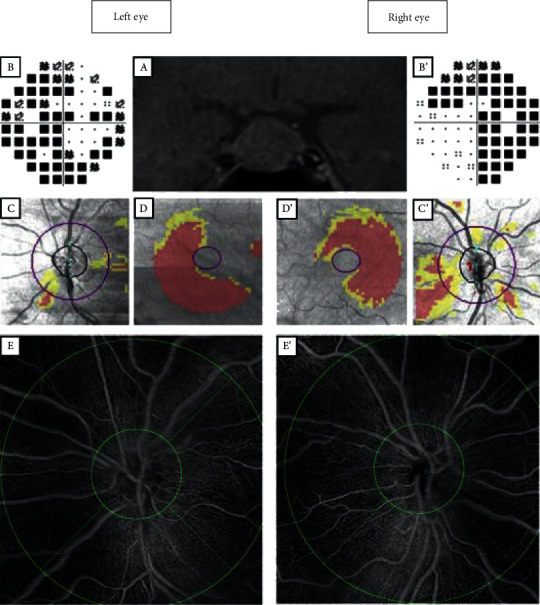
Multimodal analysis of a patient with a large pituitary adenoma with bilateral optic neuropathy. (A) Brain T1 magnetic resonance imaging showing a large pituitary adenoma with a mass effect on the optic chiasm. (B, B′) Bitemporal hemianopsia on the Humphrey visual field (30–2). (C, C′) Retinal nerve fiber layer thickness map. (D, D′) Ganglion cell complex analysis. (E, E′) “En face” optical coherence tomography angiography, 6 × 6 mm, with early treatment diabetic retinopathy study grid.
